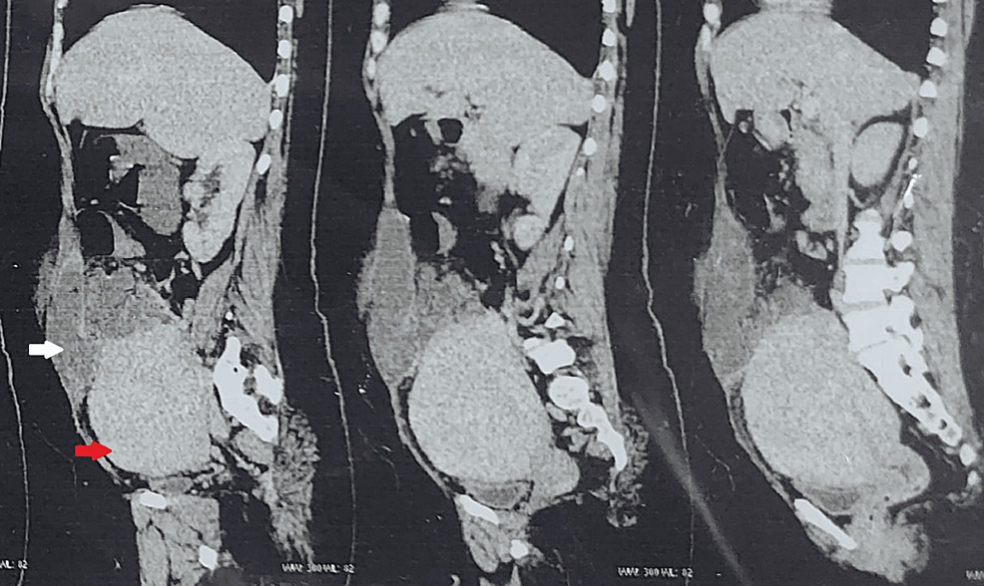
Sagittal planes of computed tomography showing ruptured tubo-ovarian abscess dissecting into rectus sheath plane (white arrow) and grossly bulky uterus (red arrow).
